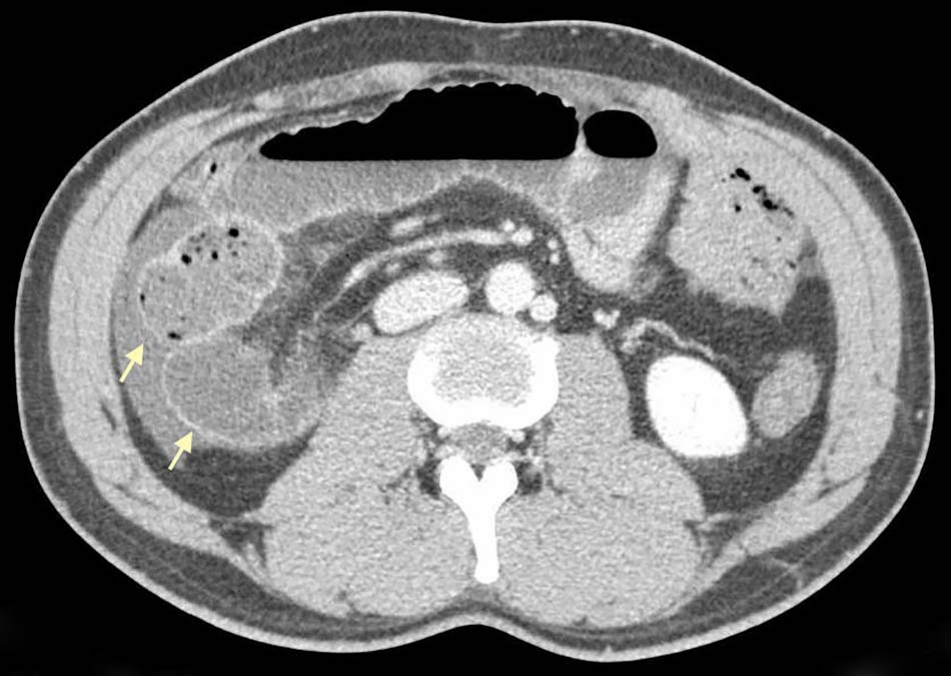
Axial CT scan shows retrocecal position of terminal ileum and free fluid in the right abdominal quadrants (arrows).
